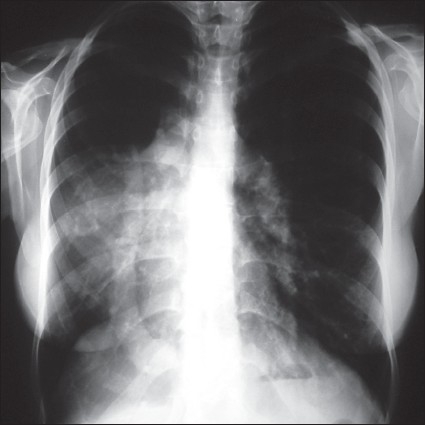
Posterior-anterior x-ray of the chest. Note that the heart is shifted into the right chest. The right lung is hypoplastic. Also note the “scimitar sign”-a curvilinear opacity widening in its course to the inferior vena cava.
